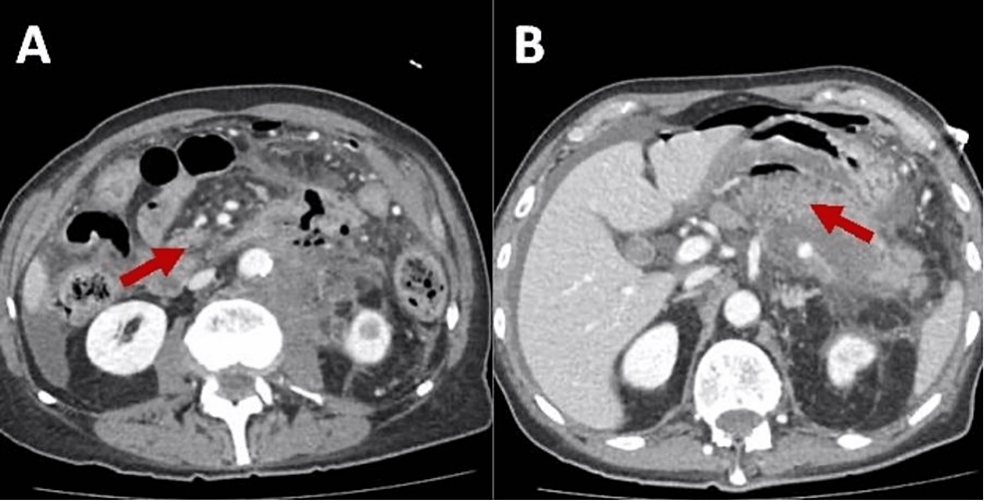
Computed tomography (CT) showing extensive complex acute pancreatitis with stable early walled-off collection and possible early necrotizing collection (A). Anterior pancreatic gastric perforation with wall thickening and second proximal jejunum perforation near peripancreatic fluid/inflammatory change (B).
